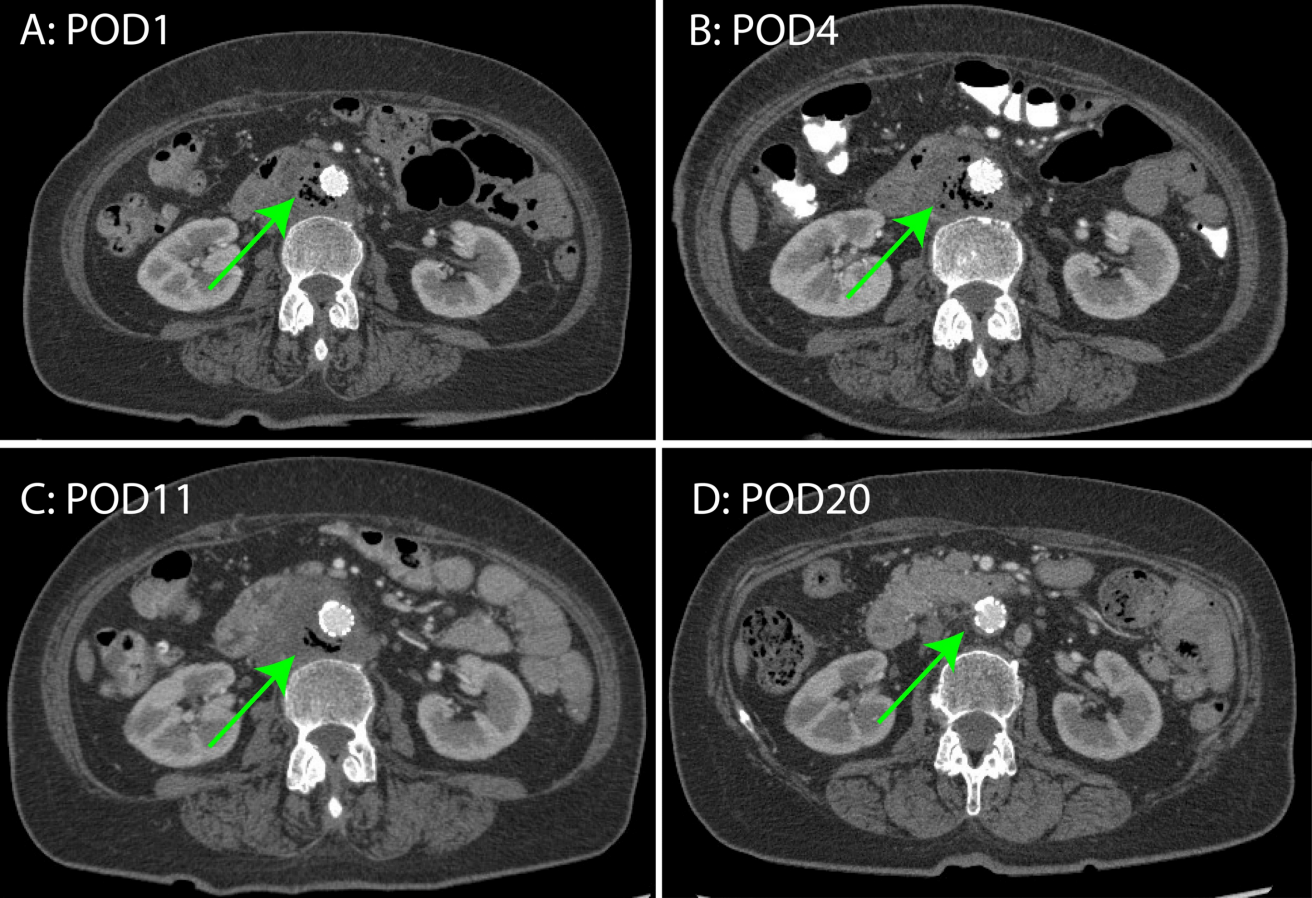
Postoperative enhanced computed tomography images (A) Postoperative day 1 (POD1). (B) POD 4. (C) POD 11. (D) POD 20. Over time, serial imaging studies revealed gradual resolution of periaortic air and complete dissipation of the inflammatory tissue surrounding the aorta (green arrows).
